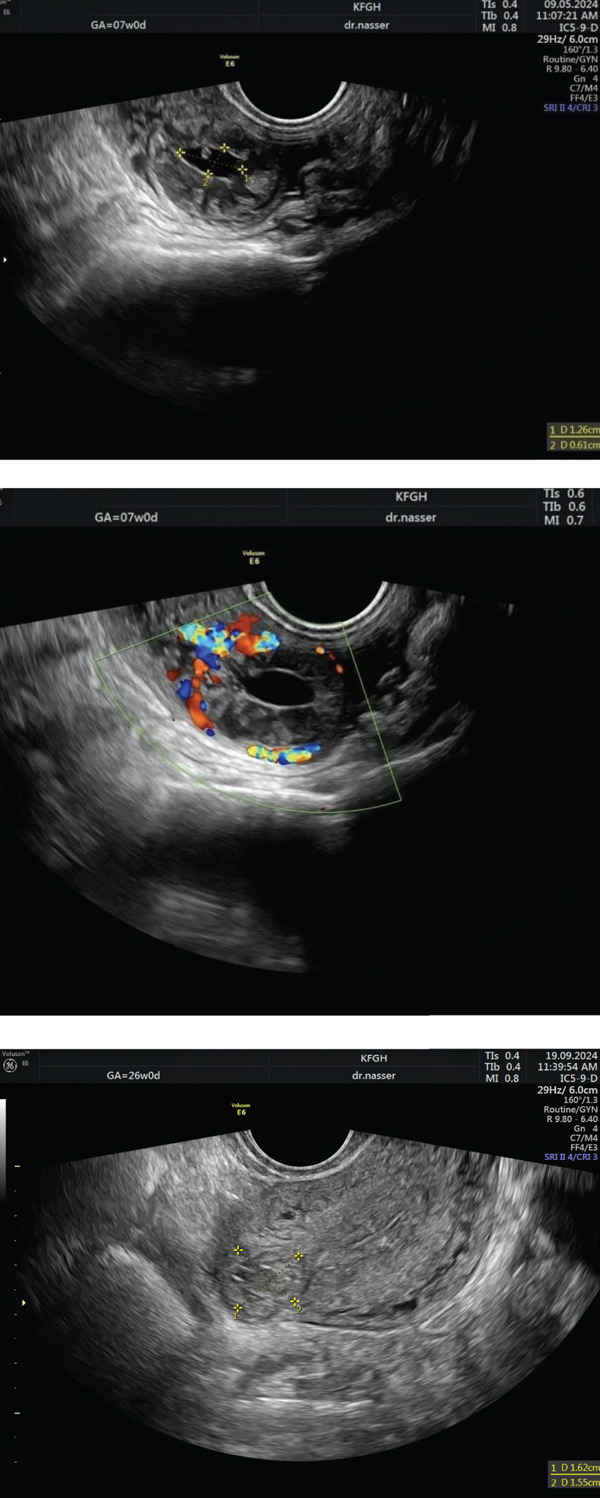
Ultrasound image of an interstitial ectopic pregnancy before the initiation of methotrexate therapy.
